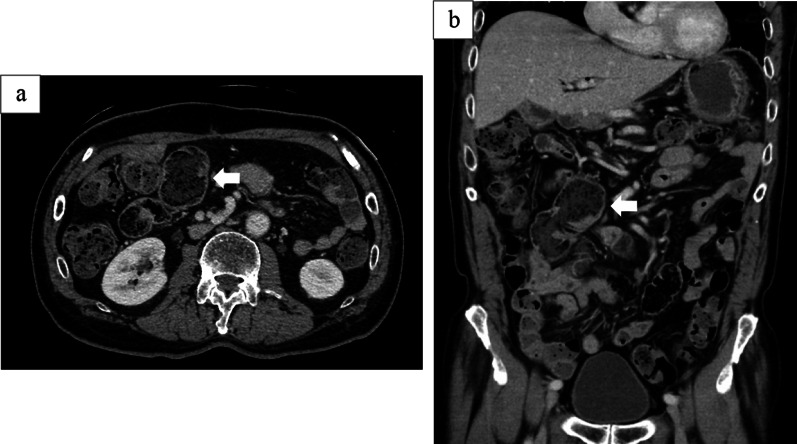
CT imaging showed dilatation of the Roux-Y-limb (white arrow)
